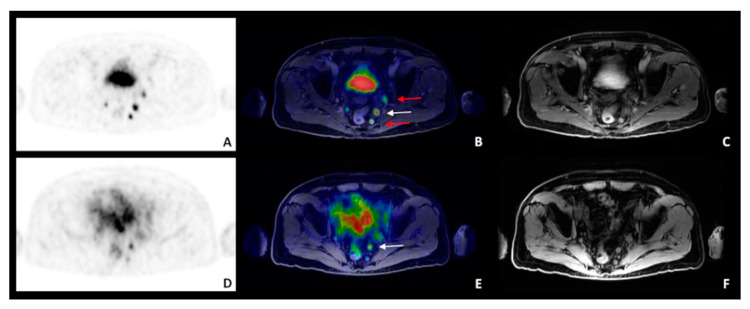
A 75 years-old patient with biopsy-proven PCa (patient n. 3), Gleason score 9 (4 + 5) with a PSA level at diagnosis of 4.73 ng/mL. ^68^Ga-PSMA and ^68^Ga-DOTA-RM2 PET/MRI were discordant in detecting lymphnodal metastases. ^68^Ga-PSMA PET/MRI (top panel; (A): transaxial ^68^Ga-PSMA PET; (B): ^68^Ga-PSMA PET/MRI; (C): post-contrast Water Lava-Flex sequence) showed bilateral pararectal and left external iliac lymphnodal uptake; ^68^Ga-DOTA-RM2 PET/MRI (bottom panel; (D): transaxial ^68^Ga-DOTA-RM2 PET; (E): ^68^Ga-DOTA-RM2 PET/MRI; (F): Water-Lava Flex sequence) showed left pararectal and left external iliac lymphnodal uptake. White arrow indicates the lymph node clearly detected by both tracers; red arrows lymph nodes detected by ^68^Ga-PSMA PET/MRI only.
